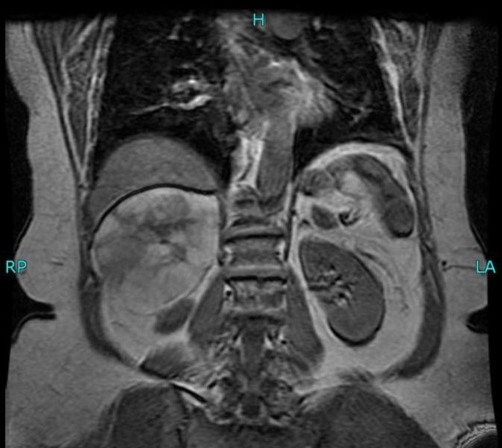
MRI confirming that the mass is an adrenal myelolipoma.
